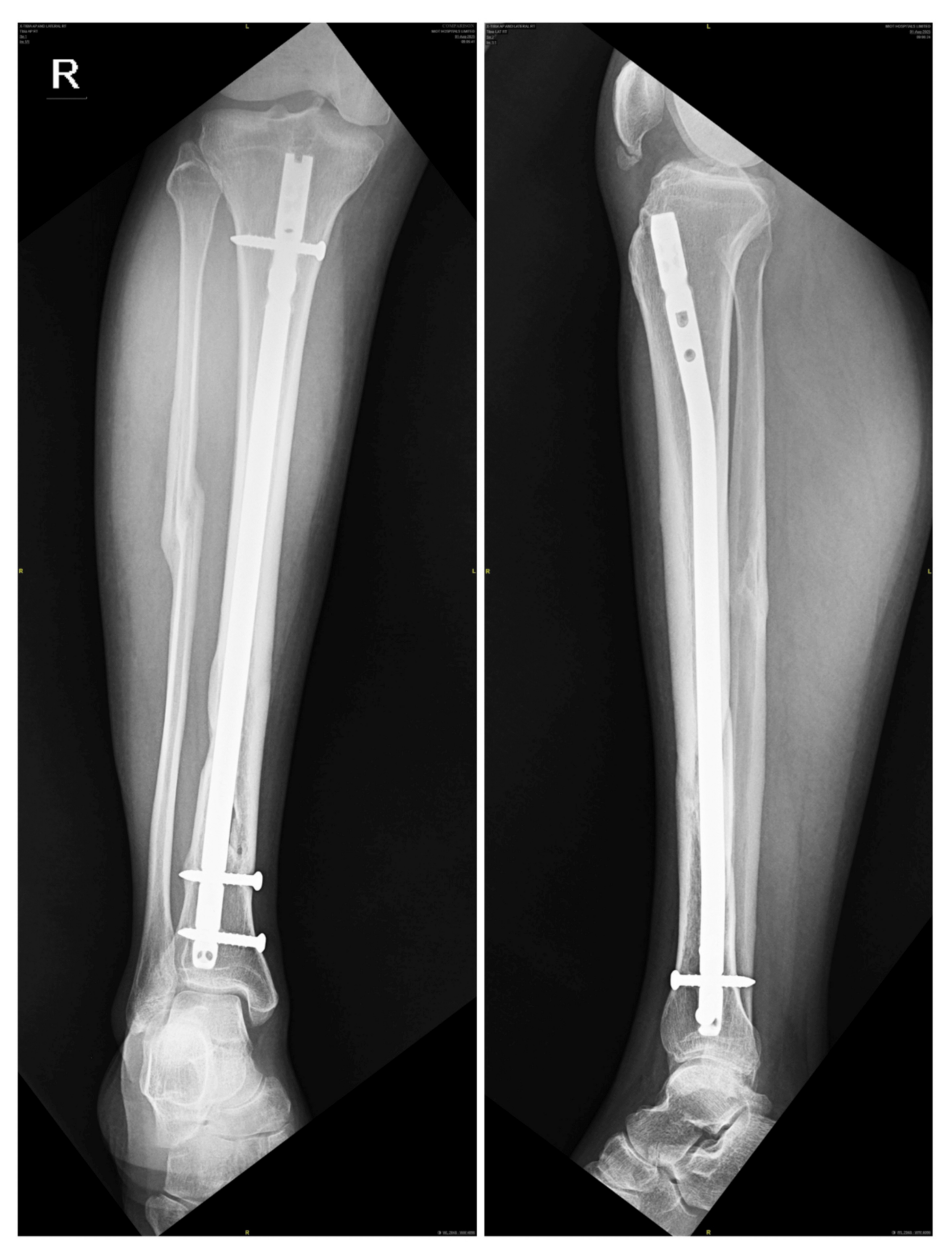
X-ray of the right tibia showing healed shaft of the tibia fracture, six years following cannulated tibial nail (10 mm in size) with one proximal locking screw in dynamic mode and two distal locking screws
